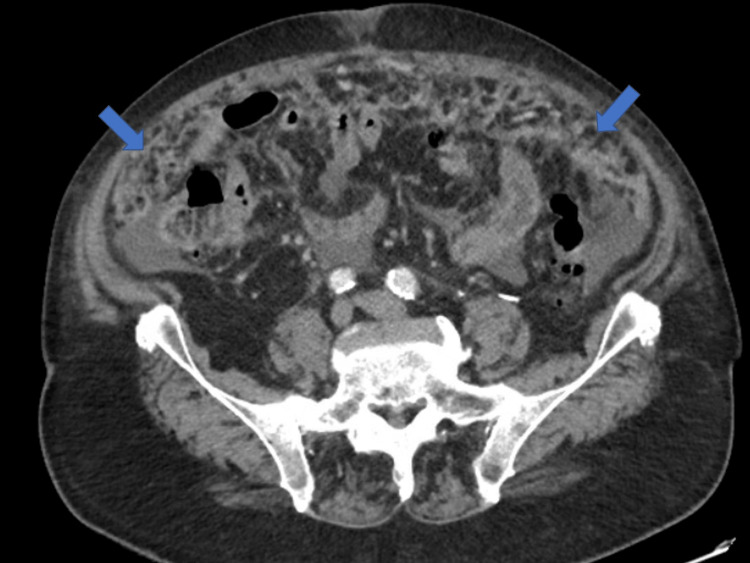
CT abdomen and pelvis with omental caking on December 22, 2020
